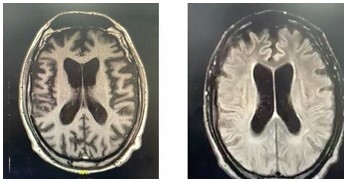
Brain Magnetic Resonance Images of the skull of patient JLS1, in which we can see global atrophy and predominance in bilateral frontotemporal regions and relative preservation of parieto-occipital regions.
